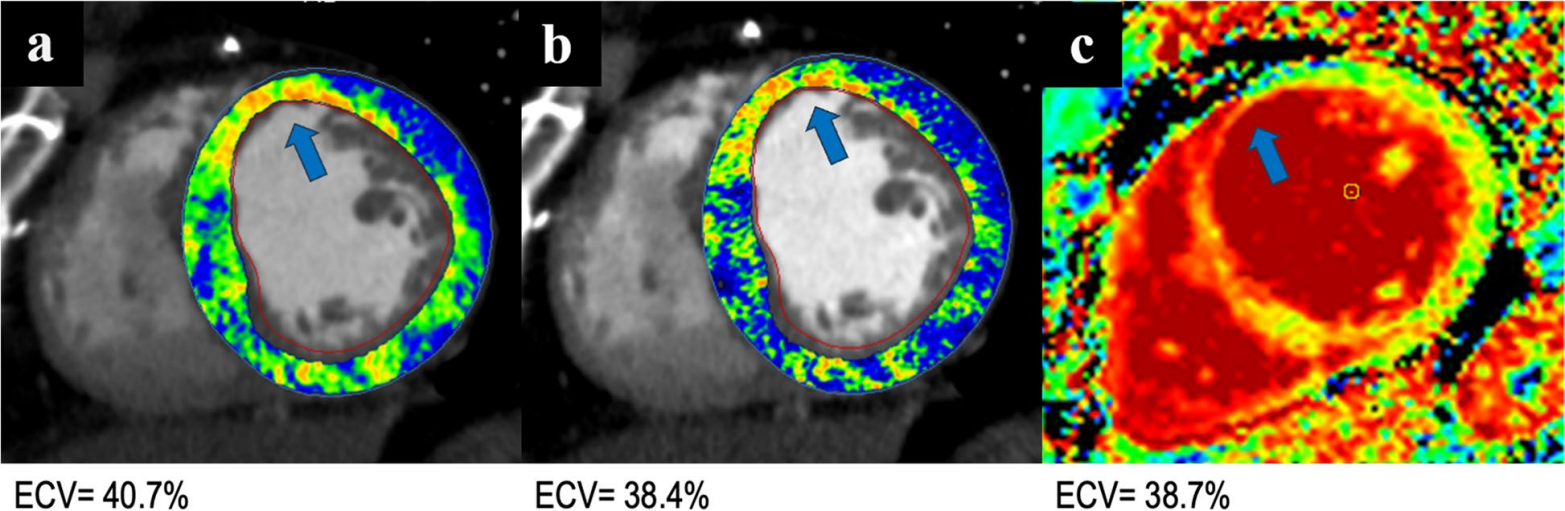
Case of a patient with a history of ischemic cardiomyopathy. CT-based ECV quantification shows anteroseptal 25−50% thickness subendocardial scar and near-transmural apical scar. The CT-based ECV quantification based on the current literature parameters (a, QIR 3, slice thickness 1.5 mm, and VMI 65 keV) overestimates the global ECV compared to the quantification performed with the most optimal combination of parameters found in our study (b, QIR 4, slice thickness 0.4 mm, and VMI 45 keV), with global ECV values of 40.7% and 38.4%, respectively, *versus* MRI-based ECV of 38.7% (c, midventricular MRI-based ECV map)
